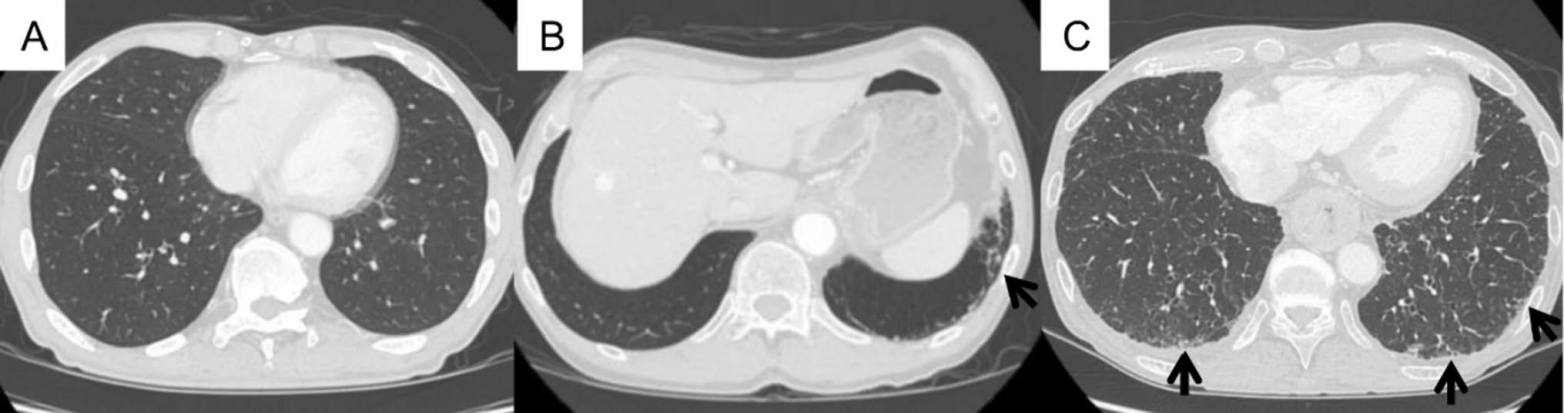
Examples of ILAs. A Non-ILA. A 63-year-old man. CT shows no interstitial lung findings. B Indeterminate for ILAs. A 71-year-old man. CT shows subtle reticular abnormality (arrow), but the lesion area is limited. C ILA. A 64-year-old man. CT shows ground-glass and irregular linear abnormalities in the subpleural areas of the bilateral lungs (arrows). *ILA* interstitial lung abnormality
